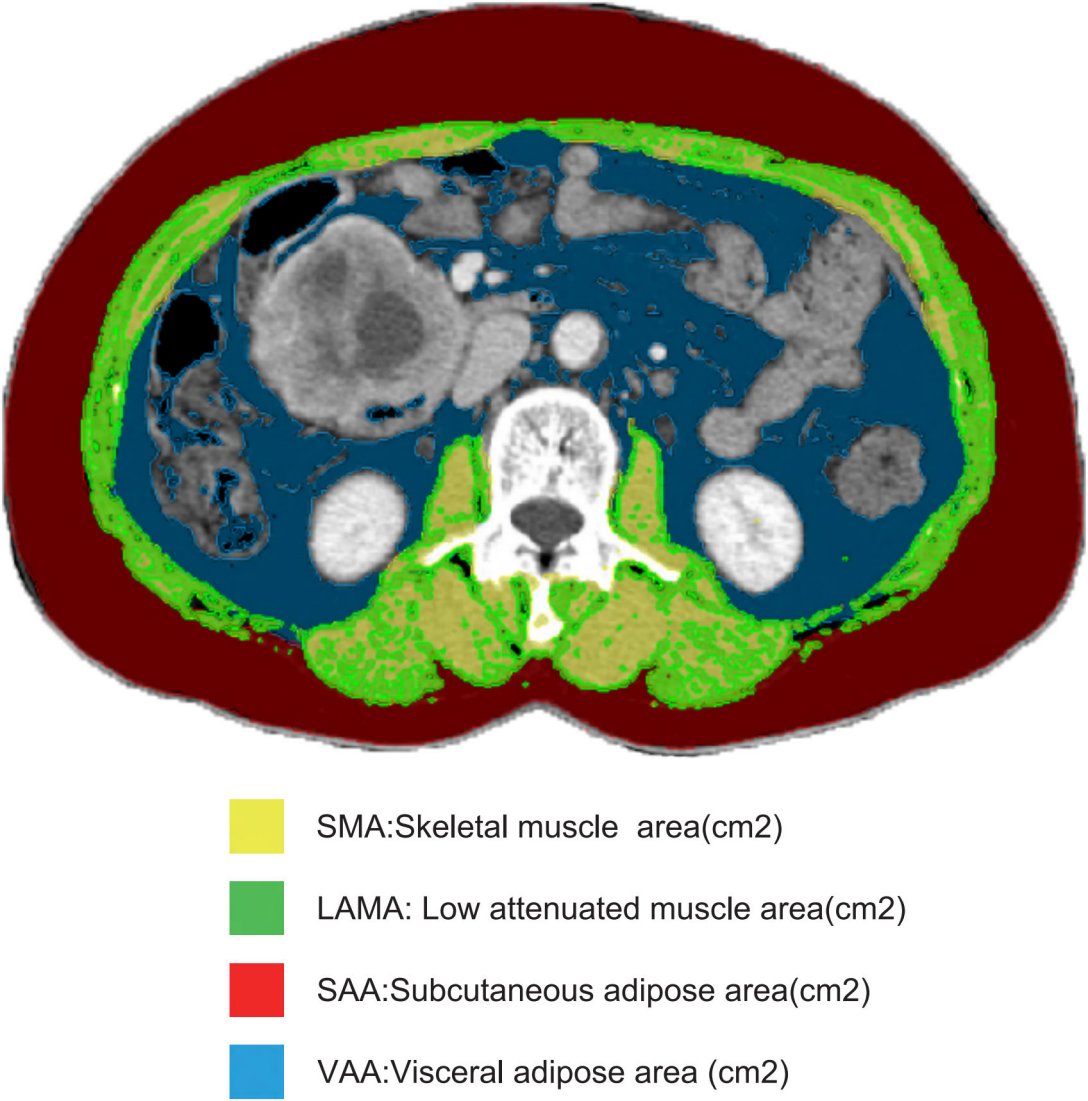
Body composition measurements. This figure presents the measurements of skeletal muscle area (SMA), low attenuated muscle area (LAMA), subcutaneous fat area (SAA), and visceral fat area (VAA) on a single CT image of the third lumbar vertebra (L3), all measured in square centimeters (cm²), used to assess body composition in the study.
